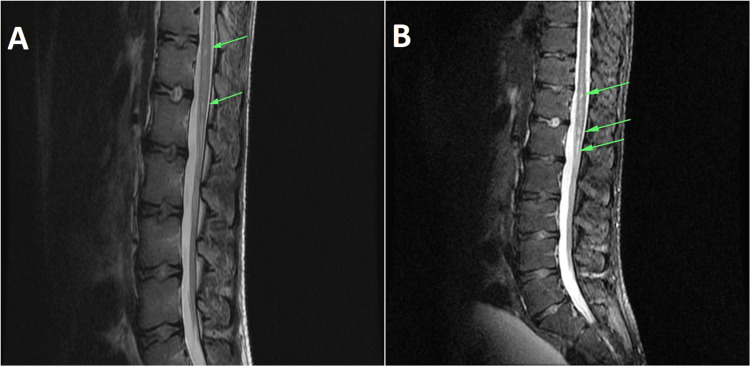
(A) T1-weighted sagittal MRI view of the thoracolumbosacral spine. (B) T2-weighted MRI of the thoracolumbosacral spine. The arrows are showing demyelination.
